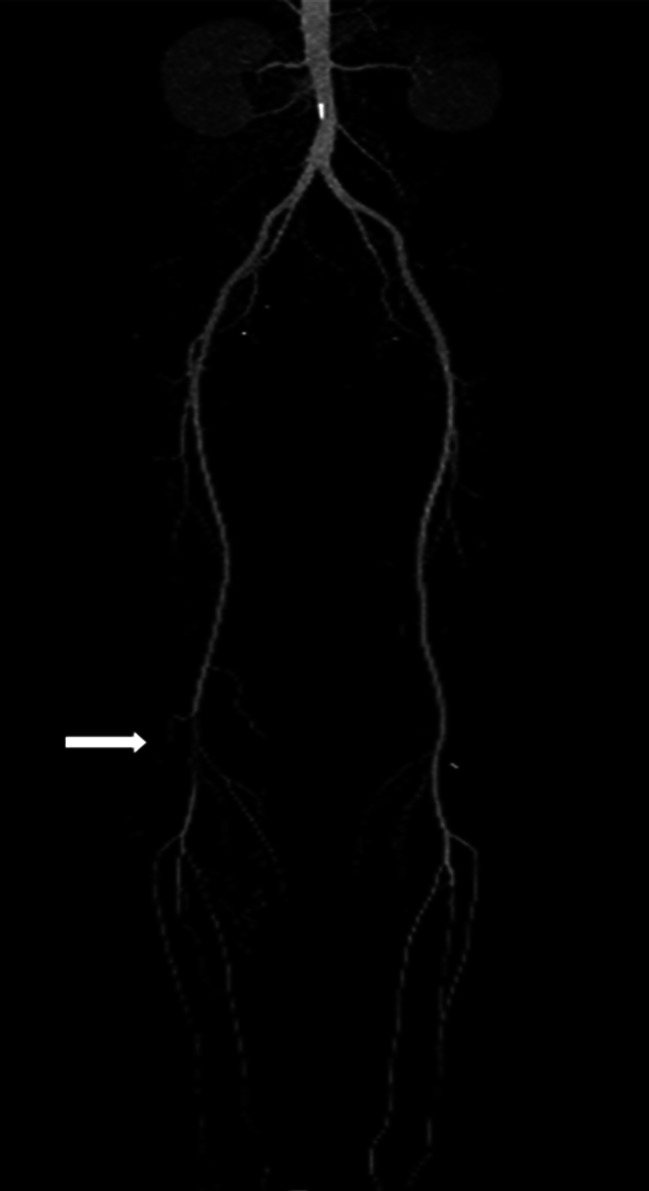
CTA showed a filling defect (arrow) in the popliteal artery due to thrombus formation
